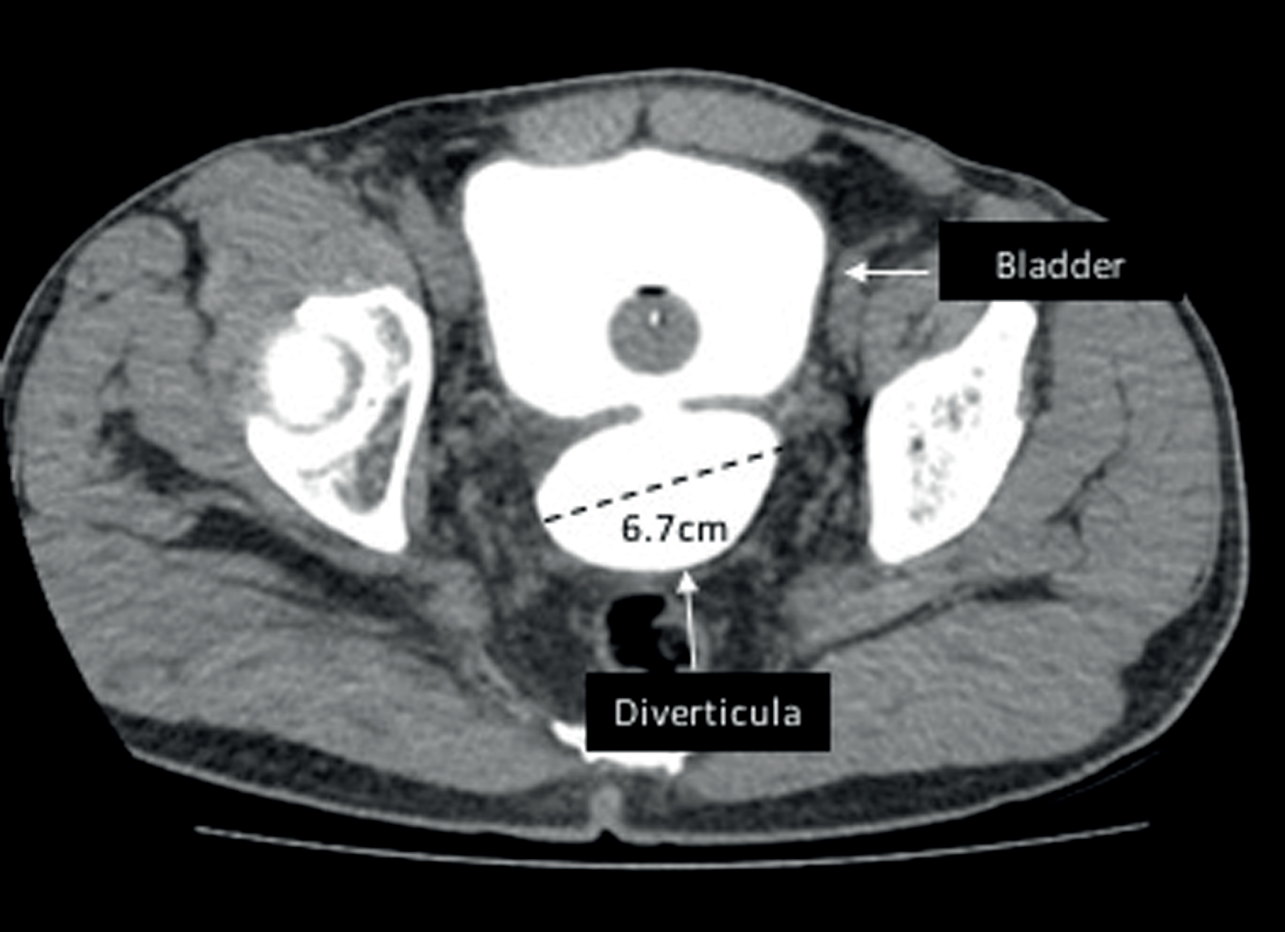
Diverticula assessment. pelvic computed Tomography (CT) with the bladder full of contrast showing posterior diverticula with 6.7cm and a narrow neck.
